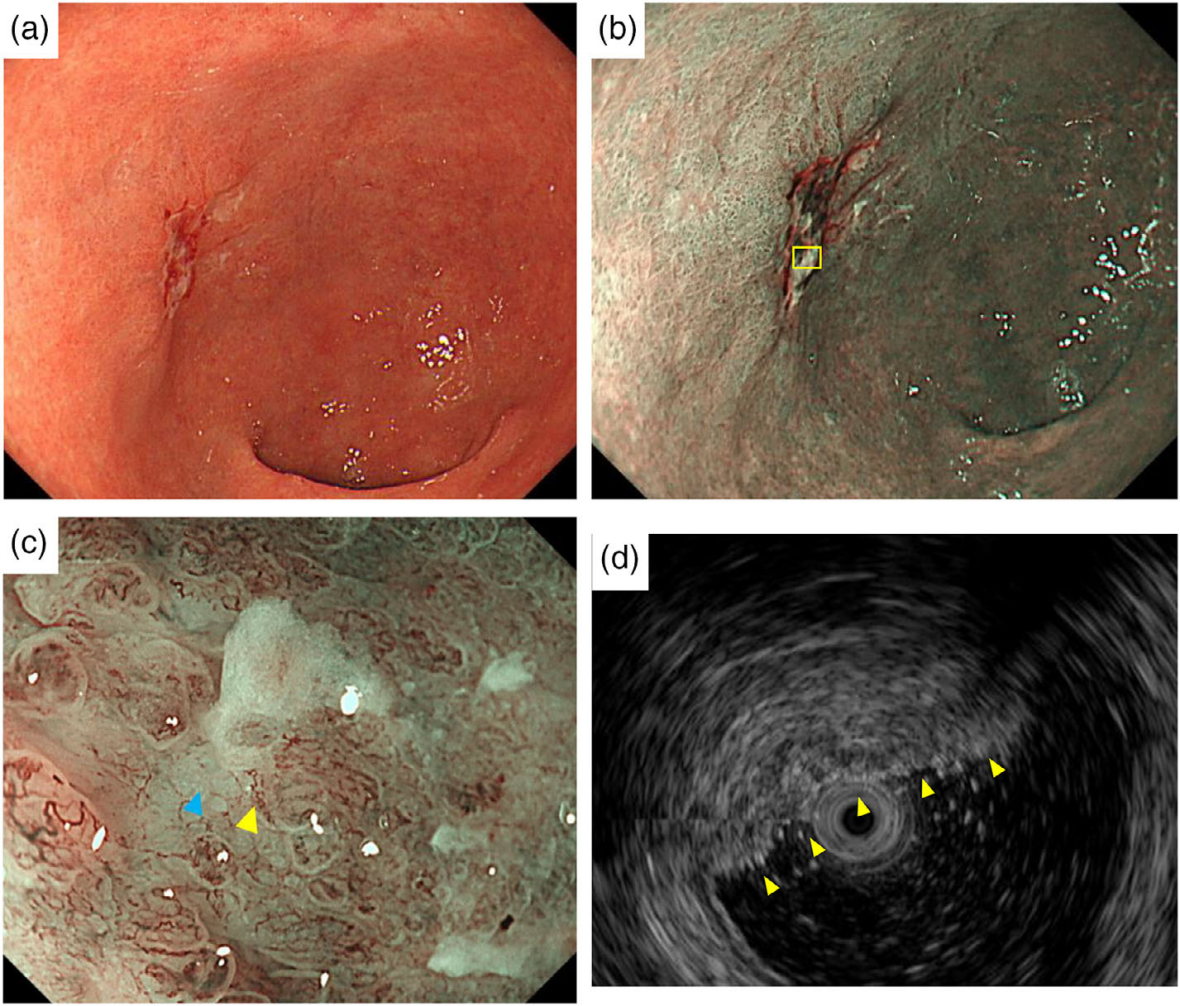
(a) A 15‐mm easily hemorrhagic 0–IIc lesion is seen near the anterior wall of the gastric antrum. The gastric wall progressed slightly well with air delivery. (b) Narrow band imaging . (c) Narrow band imaging magnifying endoscopy revealed partially obscured glandular duct structures (blue arrow) and corkscrew pattern vascular structures (yellow arrow). (d) Endoscopic ultrasonography revealed a hypoechoic mass, with no submucosal layer interruption.
